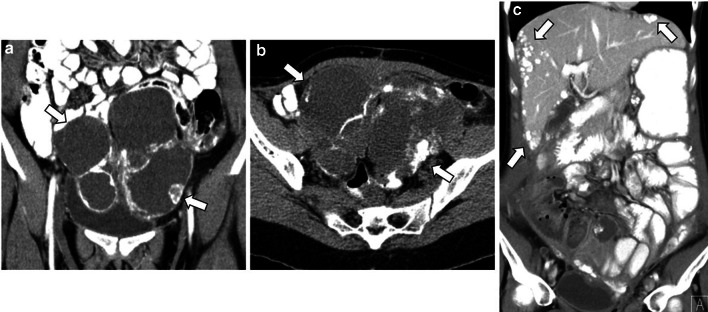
Bilateral LGSC in a 28-year-old female. Coronal and axial enhanced CT images (a, b) display a bilateral multicystic tumour with thick septa and parietal nodules with multiple psammoma bodies (arrows). Enhanced CT image (c), 3 years after cytoreductive surgery, reveals peritoneal metastases on the hepatic surface and in subhepatic space with exuberant calcified psammoma bodies (arrows)
